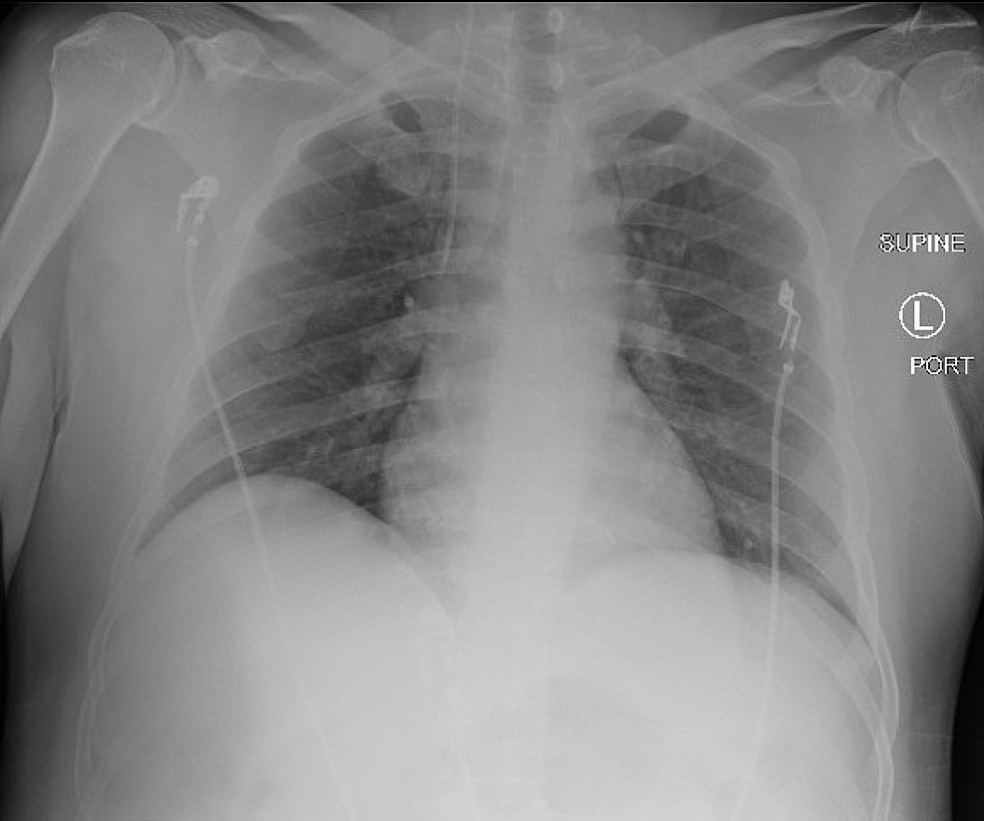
Repeat chest x-ray showed bibasilar opacities worse throughout the right lobes
